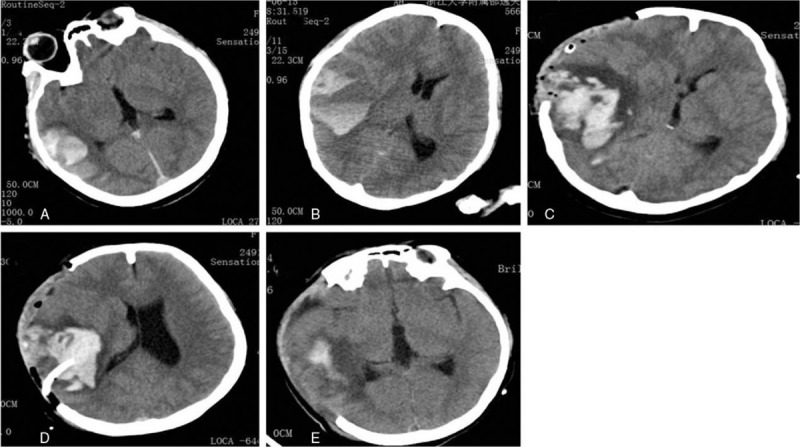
The results of all CT after mechanical thrombectomy and thrombolytics. A. Taken a CT reexamination at once after the endovascular therapy; B. Four hours after endovascular therapy; C. Two hours after decompressive hemicraniectomy; D. Two hours after the operation of punctured the hematoma supplemented by B ultrasonic; E. Two weeks after the endovascular therapy. CT = computed tomography.
